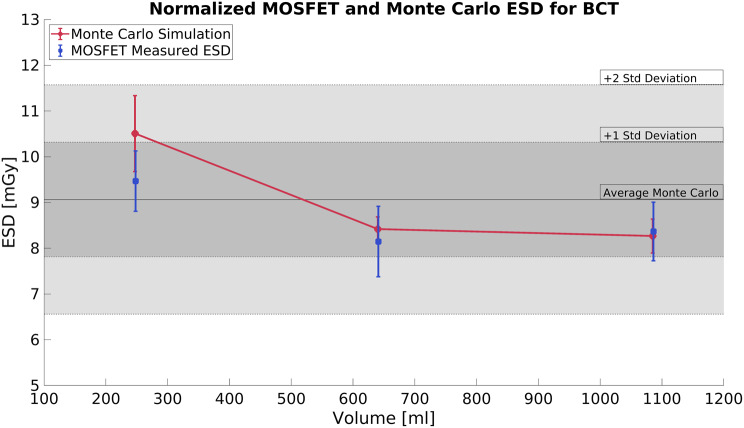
Validation of Entrance surface dose (ESD) for the breast CT data. To allow for comparison between different acquisition settings, all data points have been normalized to 25 mAs, and data for the different density mixtures have been averaged (due to the constant tube current used across different densities)
